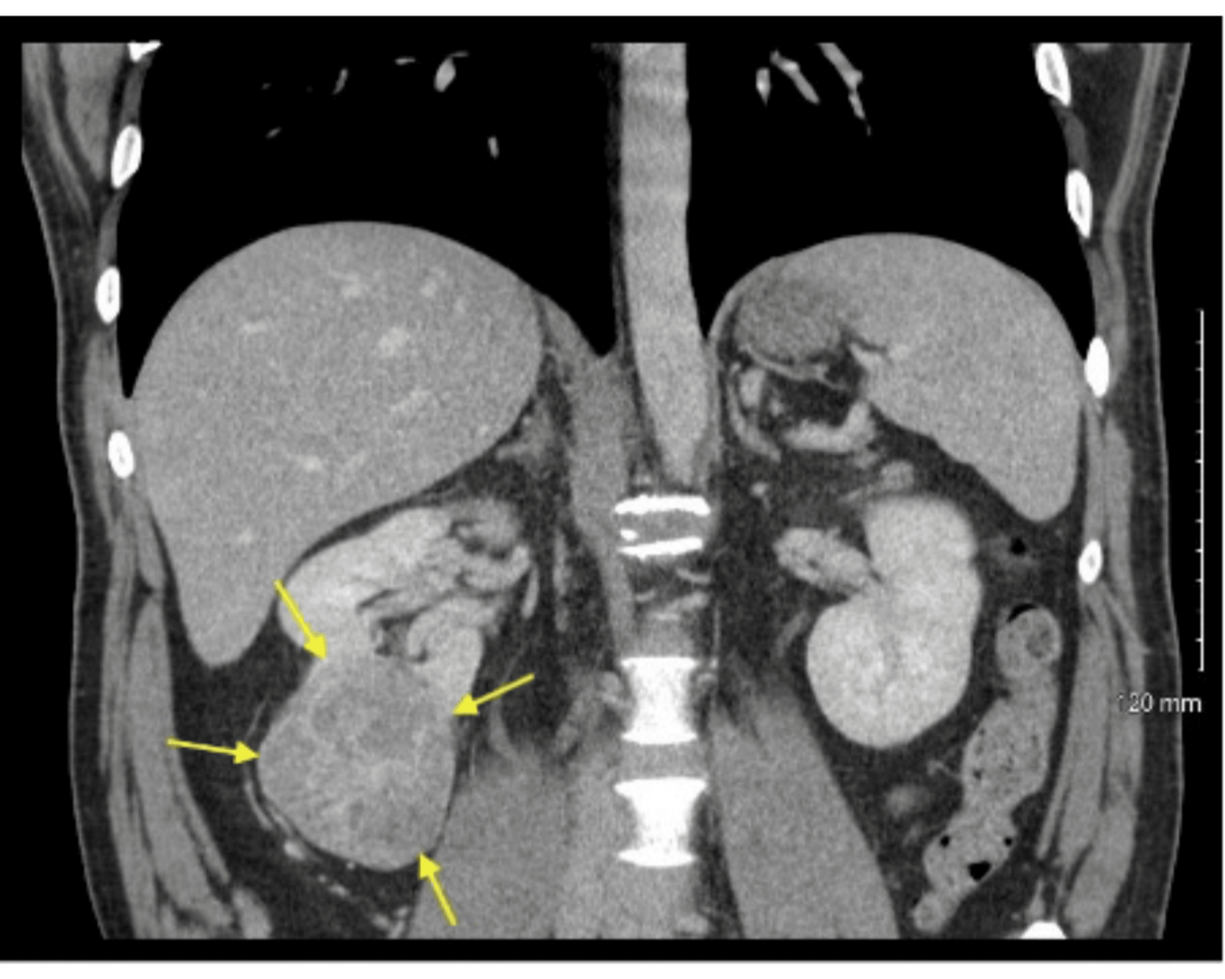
CT abdomen pelvis shows a solid mass attached to the lower pole of the right kidney (yellow arrows).
